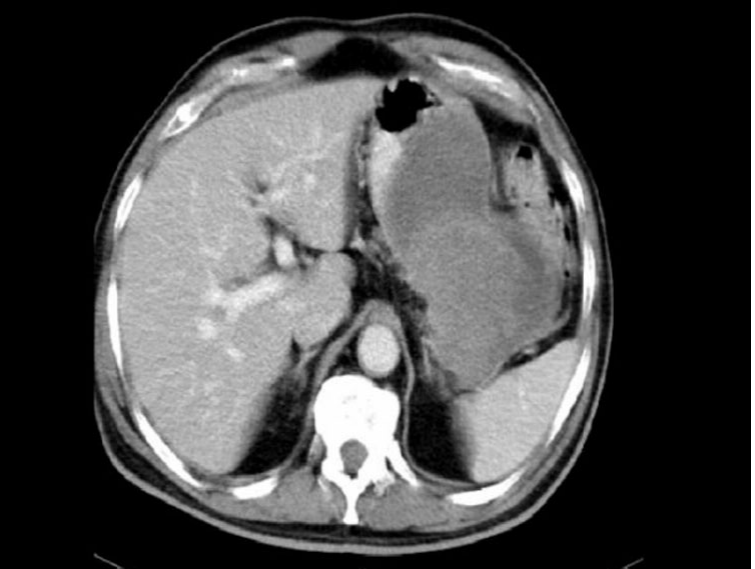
CT-Scan showed large heterogeneous mass of 13 × 10 cm size extending from the tail of pancreas to anterior prararenal space, lesser sac to gastrosplenic ligament enveloping the posterior aspect of fundus, body and greater curvature.
